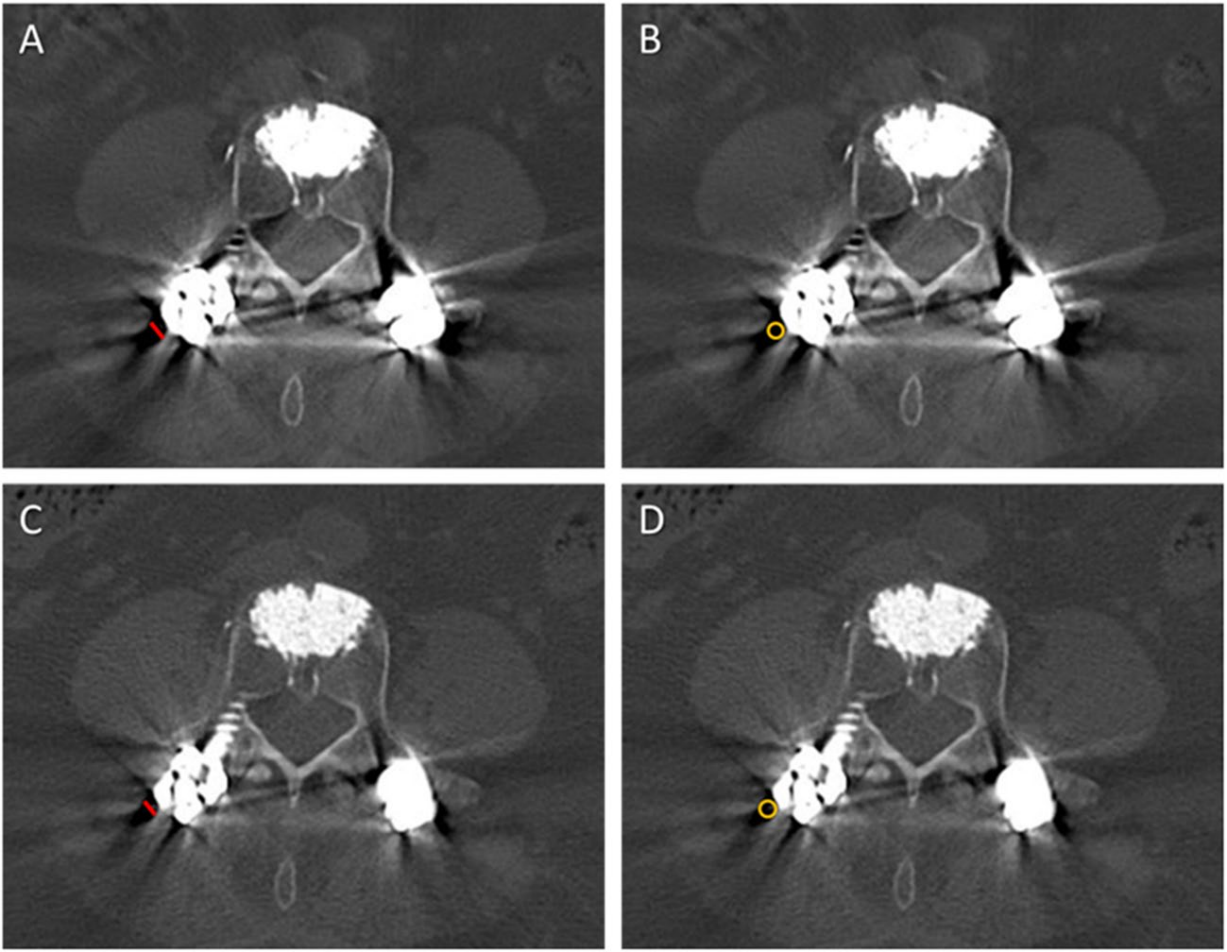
Measurements of the width and CT value of the most prominent hypodense artifact around the metallic spine implant, exemplary in a 74-year-old female with cement-augmented lumbar instrumentation. Reformatted axial CT image of the standard CT with 120 kV (A and B) and of the low-dose CT with tin filtration (Sn 140 kV; C and D) both demonstrate the measurement of the width (greatest diameter in millimeter; red line in A and C) and of the attenuation (region of interest; yellow circle in B and D) of the most prominent hypodense artifact around the metallic spine implant
